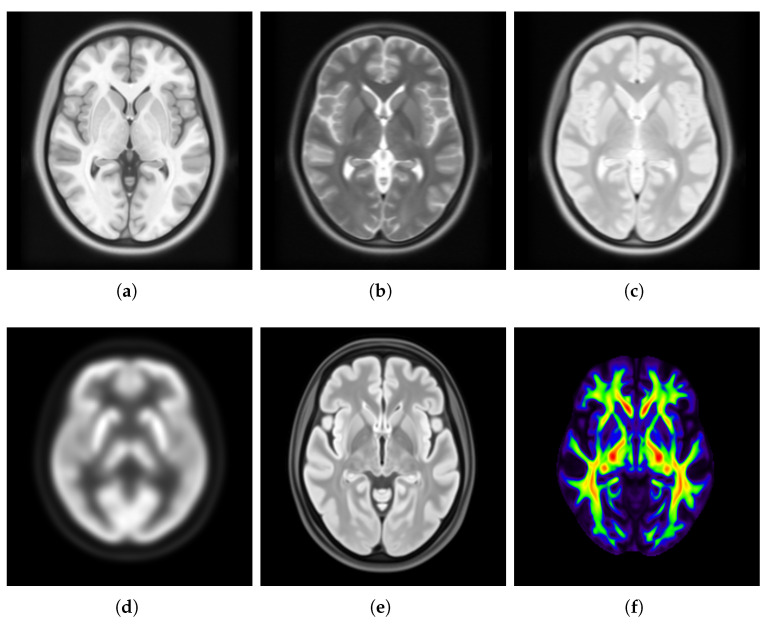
Visualization of brain templates created from various imaging modalities: (a) T1-weighted MRI, (b) T2-weighted MRI, (c) PD, (d) PET, (e) FLAIR, and (f) DTI. The grayscale contrast in (a–e) reflects the intrinsic contrast characteristics of the respective imaging modality. The colors in (f) represent diffusion directionality, visualized as a color-coded map.
